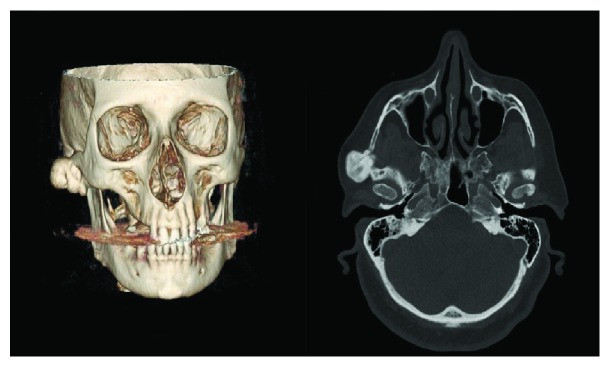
Axial CT scan and three-dimensional reconstruction image showing a well-defined, pedunculated, and radiopaque mass of the right zygomatic arch.
